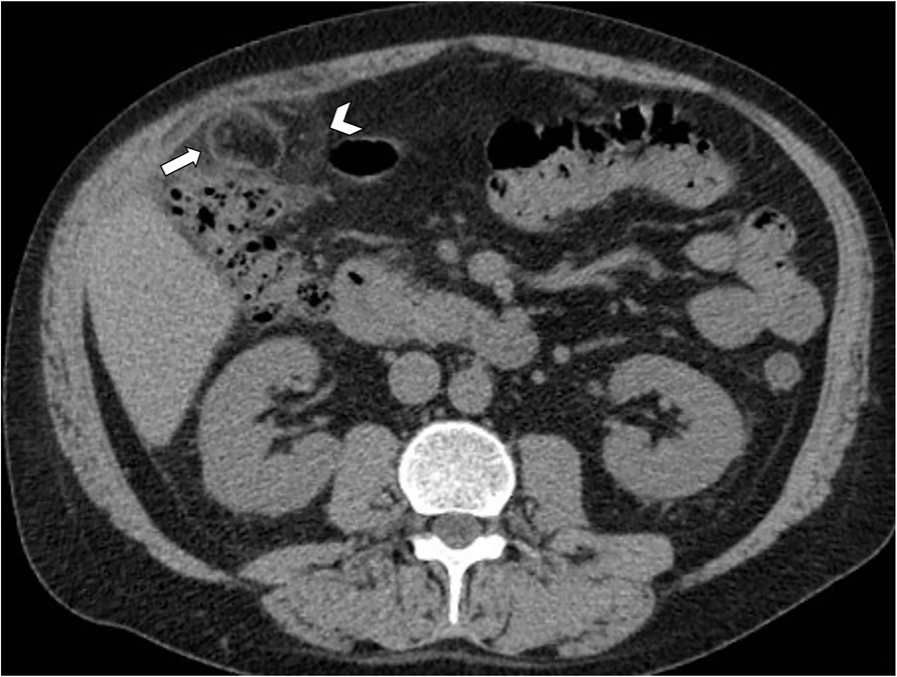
Acute epiploic appendagitis in a 53-year-old man. Axial non-contrast CT image demonstrates a fat-density lesion (arrow) that abuts the hepatic flexure of the transverse colon. Mild fat stranding is surrounding the lesion (arrowhead)
